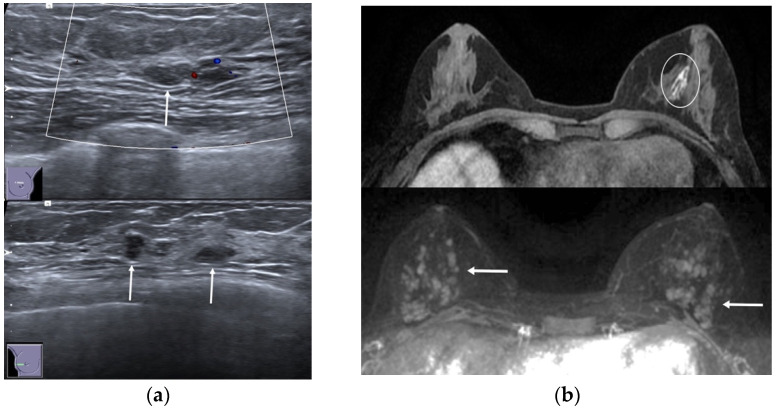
A 36-year-old woman with left bloody nipple discharge. (a) Ultrasound demonstrated bilateral multiple hypoechoic masses with circumscribed margins with ductal relation (arrows). (b) MRI showed dilated ducts with high T1 signal on pre-contrast sequences (circle) and multiple enhancing masses related with ductal contrast enhancement on post-contrast images (arrows).
